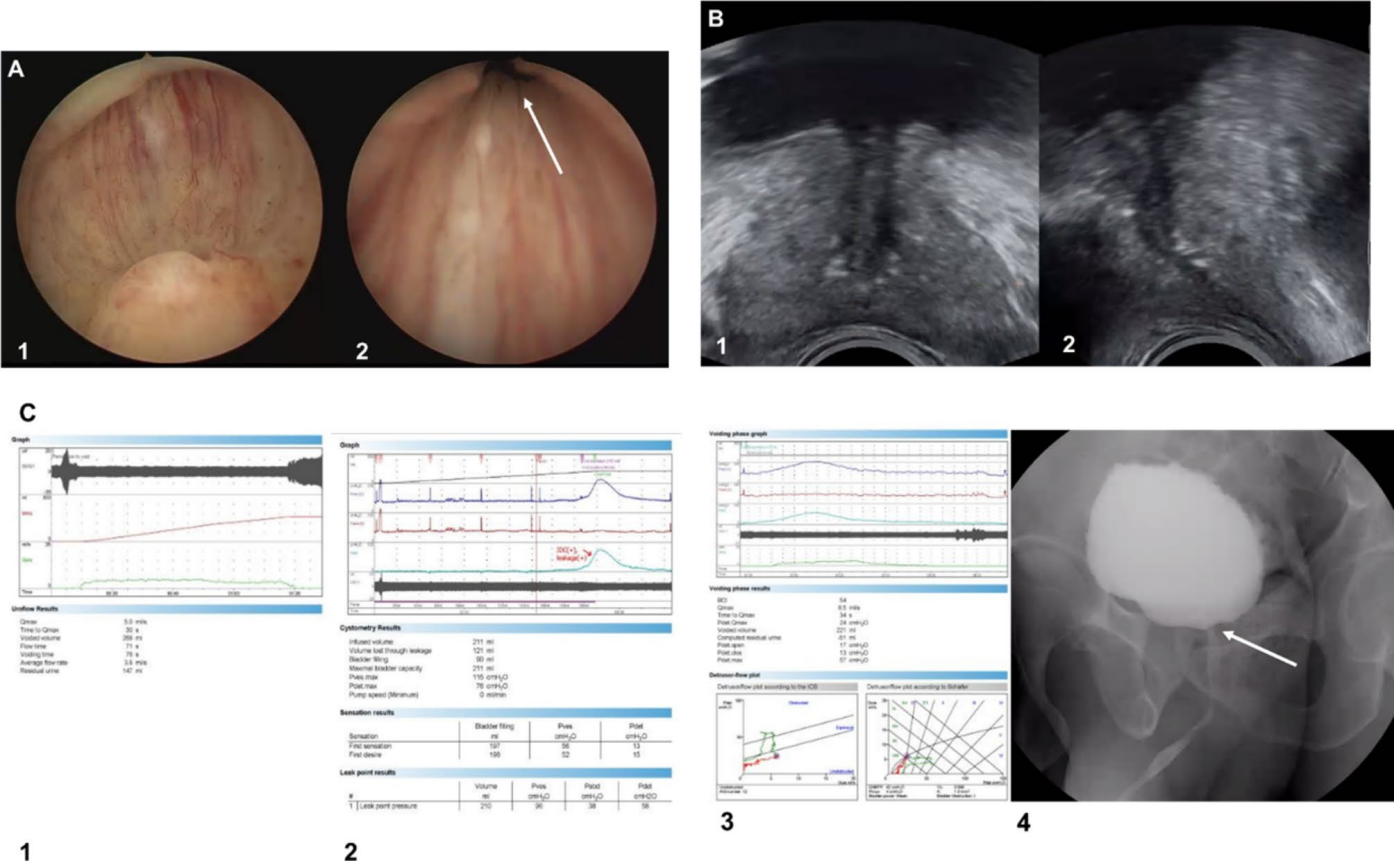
Clinical findings in a 59-year-old patient with primary bladder neck obstruction; (A) Cystourethroscopy in the lithotomy position with a horizontal view from the verumontanum shows that the bladder neck opening was not visible [1]. Bladder neck opening shows annular narrowing (2: white arrow) (B) Transrectal ultrasound imaging (1: Transverse view), (2: Sagittal view): Severe bladder neck elevation is noted in sagittal view. (C) Videourodynamic study: [1] Synnergic electromyogram (EMG) pattern of flow-EMG study and severe plateau-shaped constrictive pattern of uroflowmetry; [2] involuntary detrusor contraction on filling cystometry; [3] partly obstructive pattern of pressure-flow study; [4] bladder neck narrowing finding (white arrow) on fluoroscopic image during attempted void
